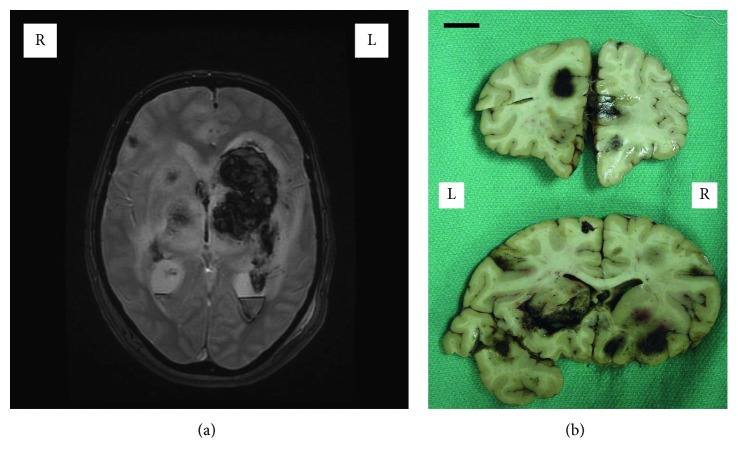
(a) Axial T2-weighted gradient echo magnetic resonance image showing hemorrhage in the left basal ganglia. (b) Gross photograph of coronal sections through the cerebral hemispheres. Scale bar = 1 cm. L = left; R = right.
